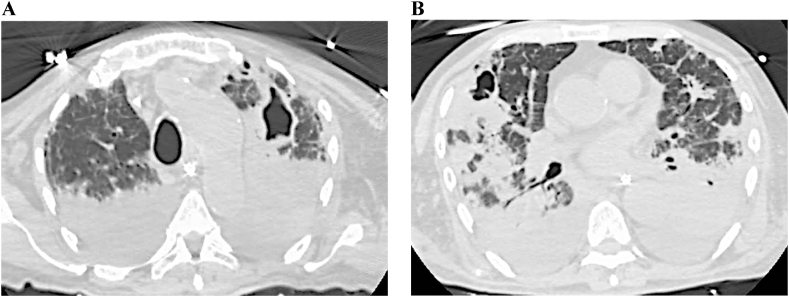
Case 3 chest HRCT. A B In the two HRTC scans (A and B) of this patient, extensive areas of lung consolidation and concomitant bilaterally excavated formations are visible.
